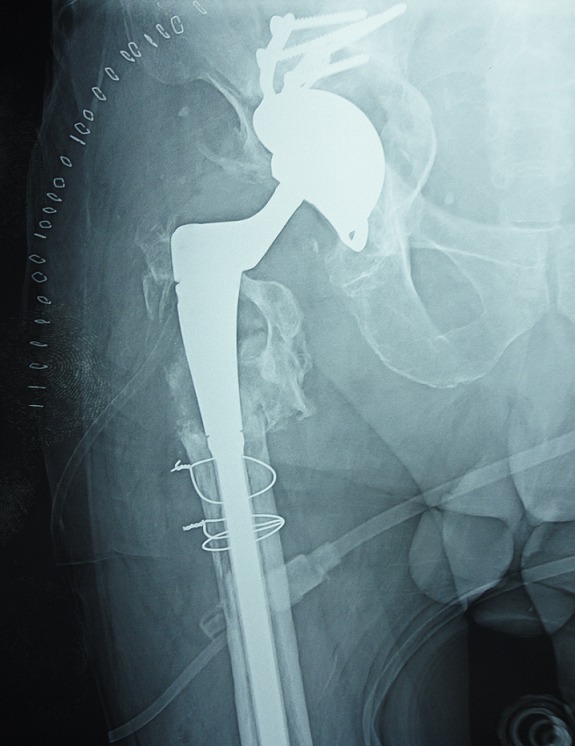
Radiographie de contrôle après reprise après 2 mois
